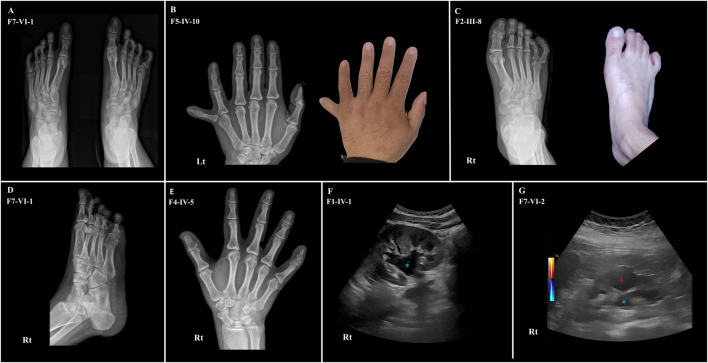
Representative images for hand x-ray, foot x-ray, selected clinical photographs of persistent polydactyly, and urinary tract ultrasound images. (A) Lt foot X-ray showing residual ossicle on the left fifth toe, resembling previous polydactyly, and a biphalangeal fifth toe. Rt foot X-ray showing clinodactyly of the right fifth toe. (B) Left hand x-ray and corresponding clinical photograph showing post-axial polydactyly of the Lt fifth finger. The sixth finger consisted of two phalanges. (C) Right foot x-ray and corresponding clinical photograph showing post-axial polydactyly of the Rt fifth toe, with the sixth toe consisting of two phalanges and clinodactyly of the fifth toe. (D) Bony small exostosis observed at the head of the fifth metatarsal bone, best appreciated on the oblique image. (E) Rt fifth finger clinodactyly, with a short, small middle phalanx. (F) Longitudinal Rt renal ultrasound image showing Rt moderate hydronephrosis (blue asterisk). (G) Longitudinal Rt renal ultrasound image showing moderate hydronephrosis (blue asterisk) and simple right renal cyst 3.2 × 2.6 cm (red asterisk). The corresponding patient IDs are shown in the upper left corner of each panel. Abbreviations: Rt, right; Lt, left.
